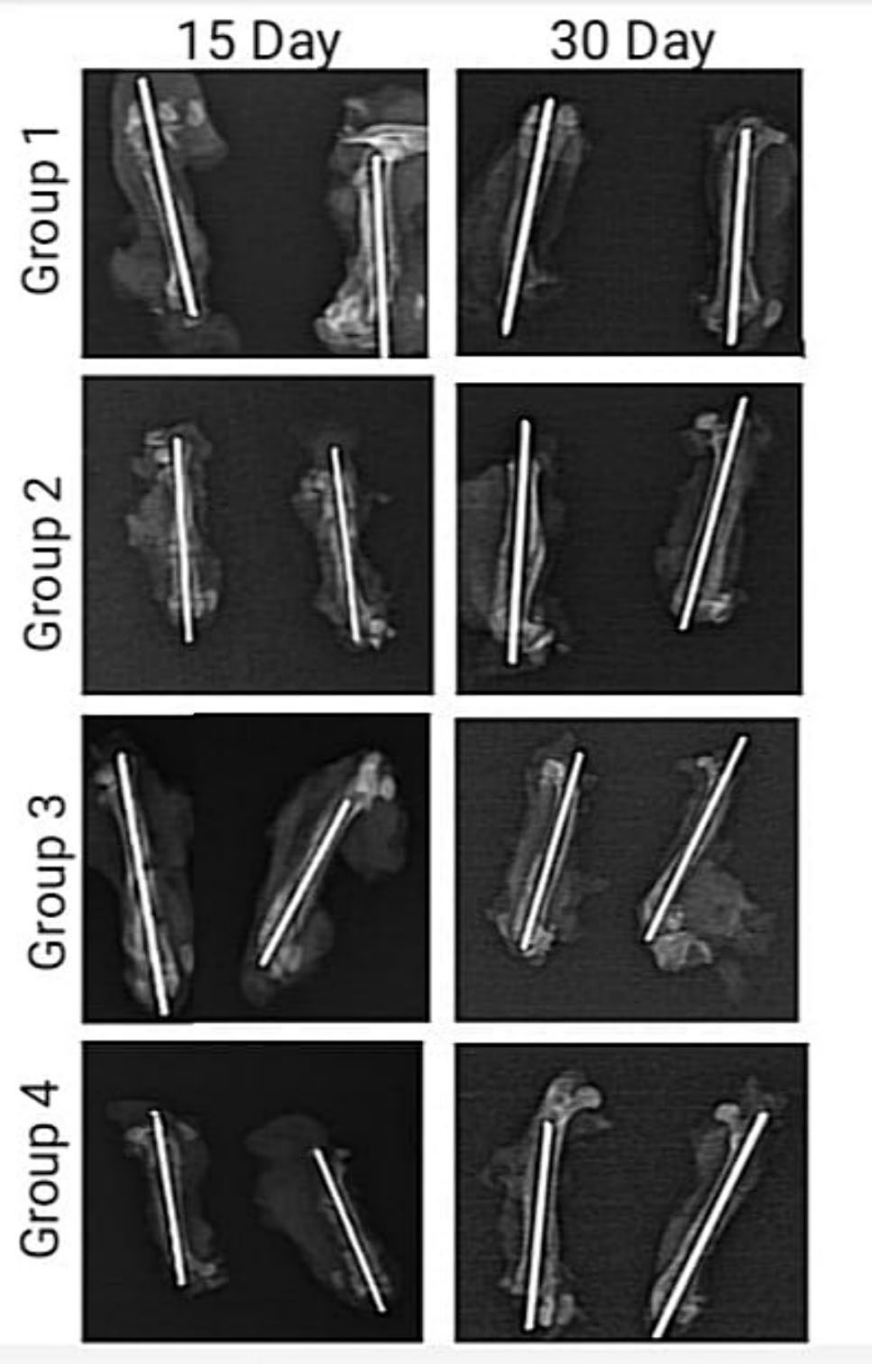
Control radiographs after surgery
